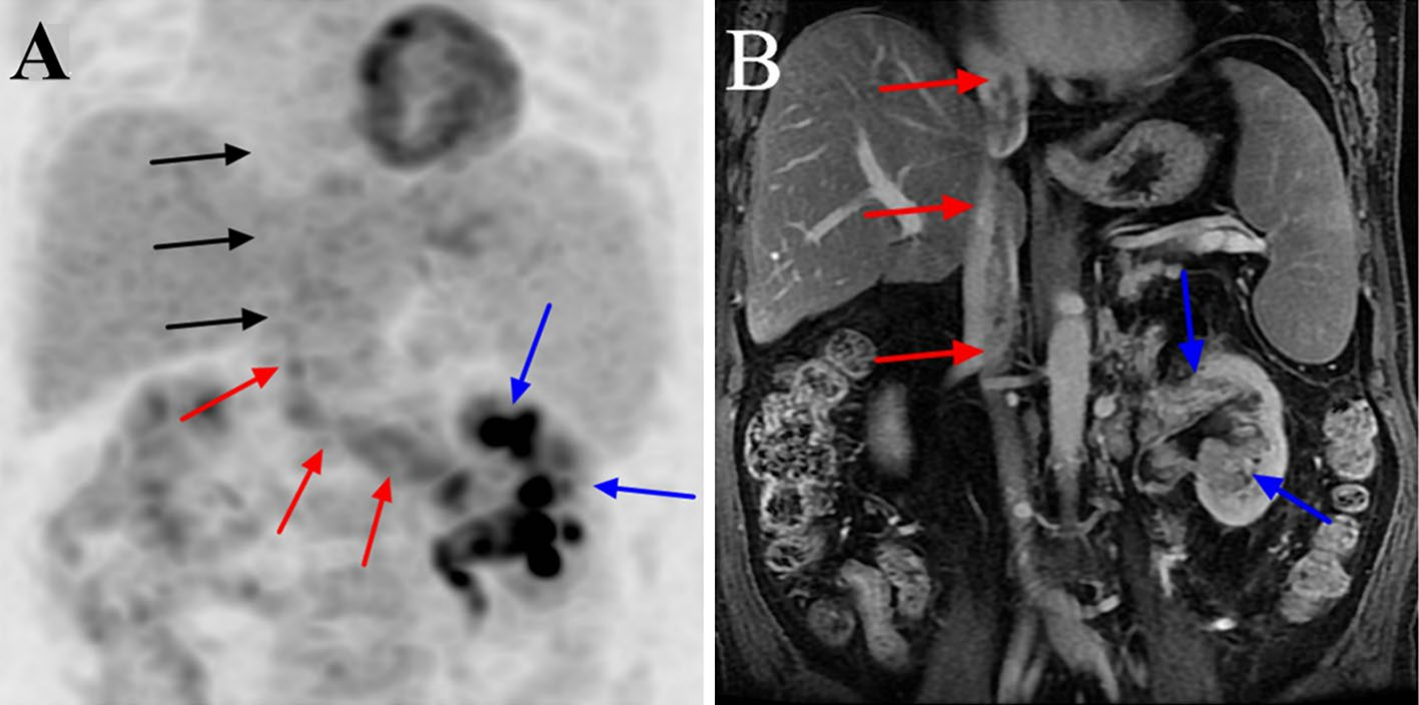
^18^F-FDG PET/CT and contrast-enhanced MRI (CEMRI) scans of a 62-year-old man with clear cell carcinoma of the left kidney associated with a level IV venous tumour thrombus (VTT). The VTT did not invade the venous wall and was graded as level II by PET/CT and level IV by CEMRI. The site of diffusely increased FDG uptake (SUVmax 6.3) on a PET maximum intensity projection image is the primary tumour (blue arrow) (a). The VTT showed pathologically increased FDG uptake (SUVmax 3.4) from the left renal vein to the inferior vena cava below the hepatic vein (red arrow) (a). However, there was no increased FDG uptake (SUVmax 2.0) from the hepatic vein to the inferior vena cava above the diaphragm (black arrow) (a). Coronal CEMRI showed that the VTT had an irregular shape, was heterogeneously enhanced, and partially occluded the vein lumen (red arrow) (b). The primary tumour also showed heterogeneous enhancement (blue arrow) (a).
